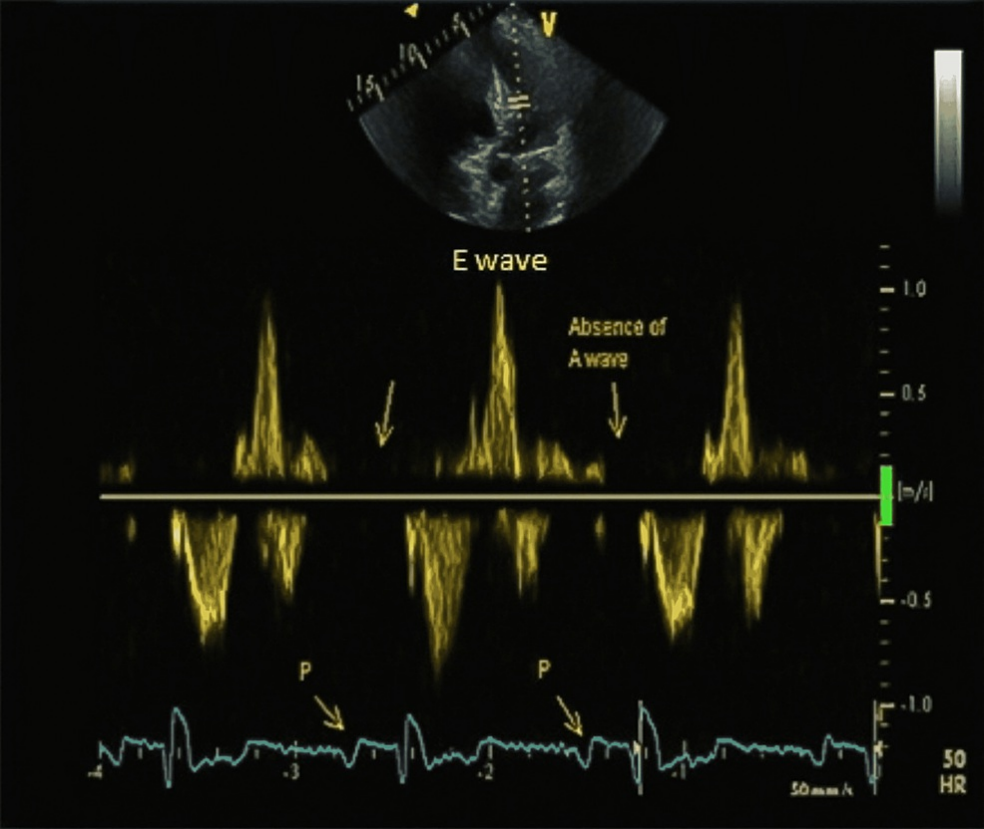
Pulsed-wave Doppler TTE showing the presence of E wave velocity with the absence of A wave (atrial contraction indicated by arrow) TTE: transthoracic echocardiogram
